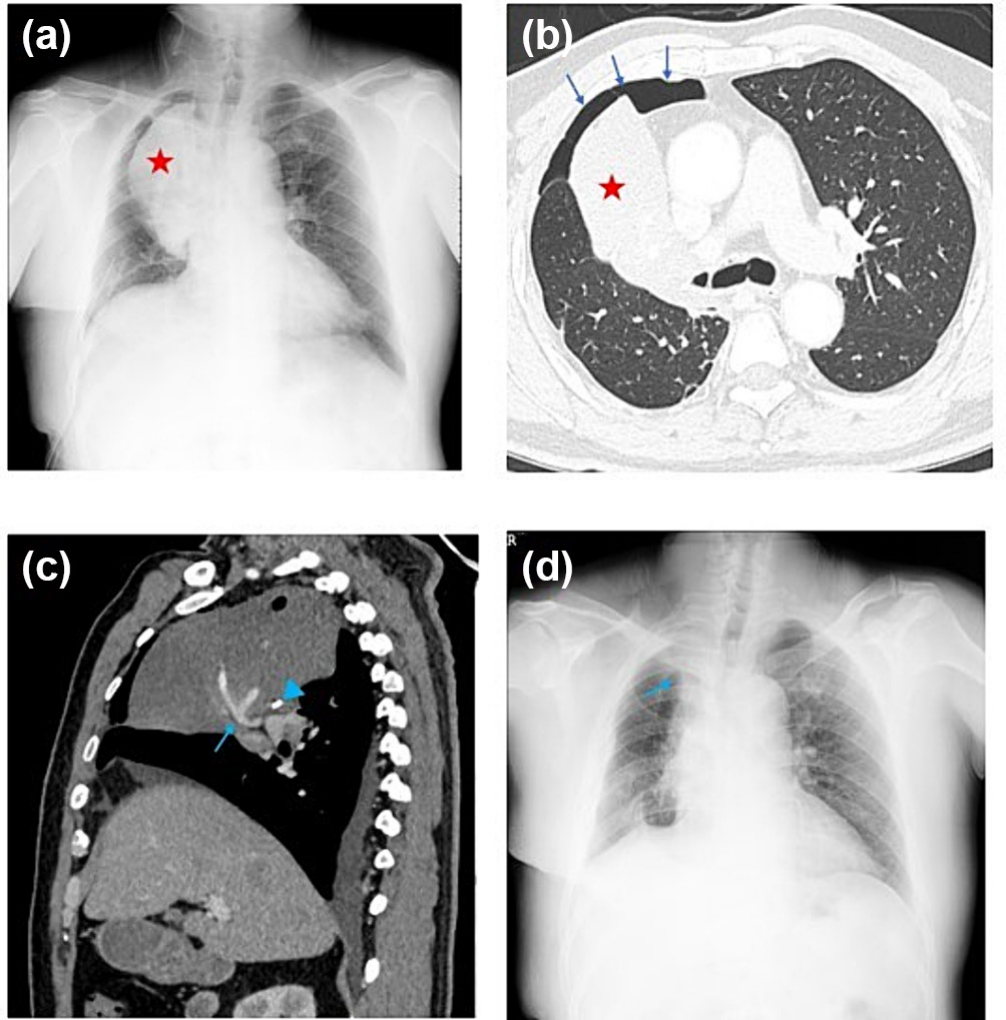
Right middle lobe torsion in a 61‐year‐old man who had undergone right upper lobectomy for invasive adenocarcinoma. (a) Chest radiograph after right upper lobectomy shows right perihilar opacification (red star) with well‐defined margin, a right chest drain, and a right central catheter. (b) Axial image of the postoperative contrast‐enhanced computed tomography (CT) scan on lung window. The middle lobe tapes towards the hilum with complete consolidation (red star). Note also the postoperative pneumothorax (blue arrows). (c) Sagittal reformat of postoperative contrast‐enhanced CT scan on soft tissue window. A tortured pulmonary artery (blue arrow) and hilar surgical sutures (blue arrowhead) can be observed. (d) Chest radiograph after the secondary operation shows increased density (blue arrow) adjacent to the right hilum but no lobar parenchymal consolidation.
